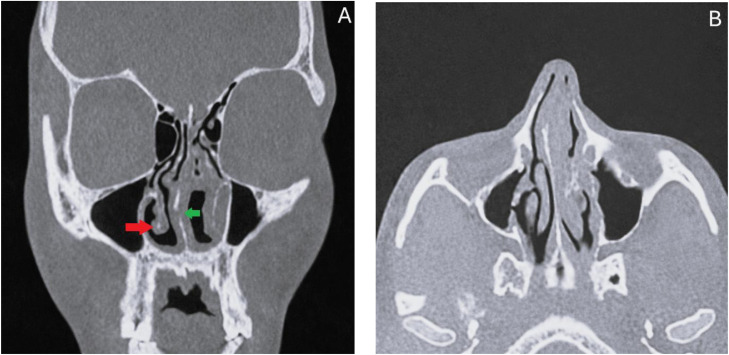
(A) Coronal head and neck CT scan (bone window) demonstrating a well-defined lytic defect involving the cartilaginous portion of the nasal septum (green arrow), consistent with focal septal lysis, associated with bilateral inferior turbinate atrophy (red arrow). The margins of the septal defect appear irregular but clearly delineated, without extension to the bony septum. (B) Axial contrast-enhanced CT scan showing leftward septal deviation with focal thickening of the septal mucosa. No abnormal soft tissue mass or contrast enhancement is identified.
